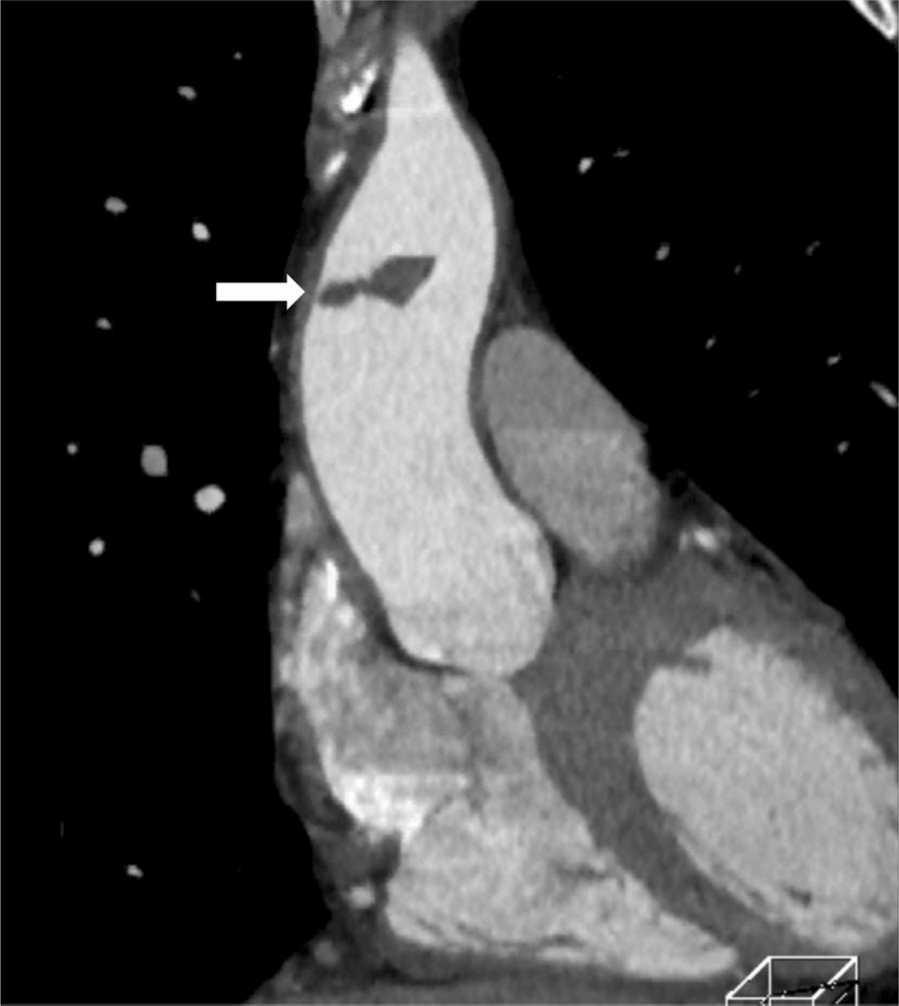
Computed tomography (CT) shows floating thrombosis (white arrow)
